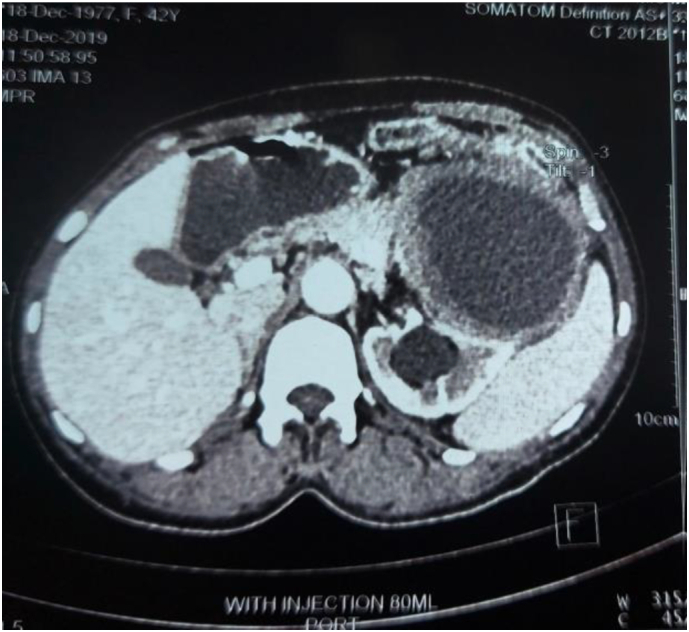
Transverse section of abdominal CT scan indicating a thick-walled, well-defined, hypodense, cystic lesion attached to the tail of pancreas.
